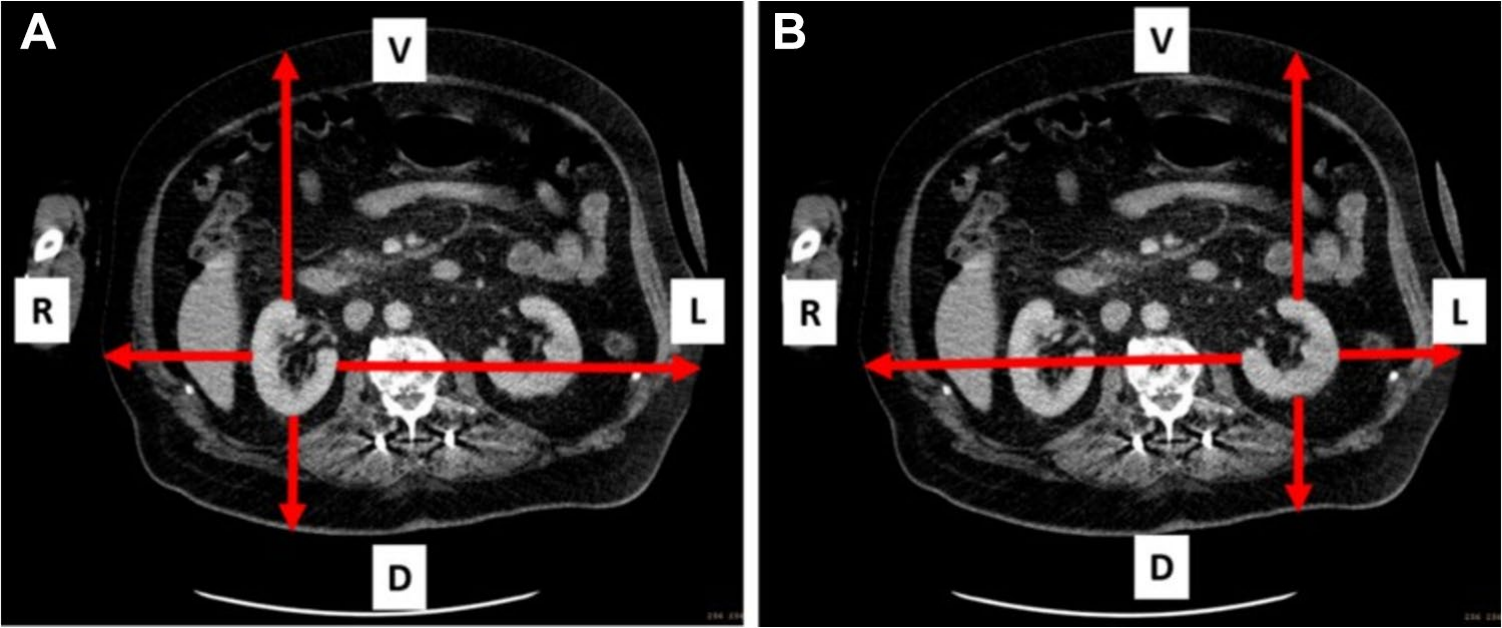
Transaxial CT-view of a 130 kg patient with measurement of the distances from the right kidney (A) and the left kidney (B) to the corresponding body surface
